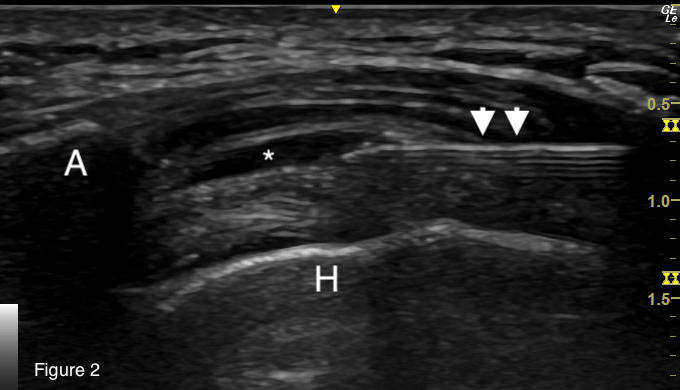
Ultrasound still image demonstrating injection into the subacromial/subdeltoid bursa. (A: acromion; H: humeral head; arrowheads: needle; asterisk: subacromial/subdeltoid bursa distended with injectate.)
